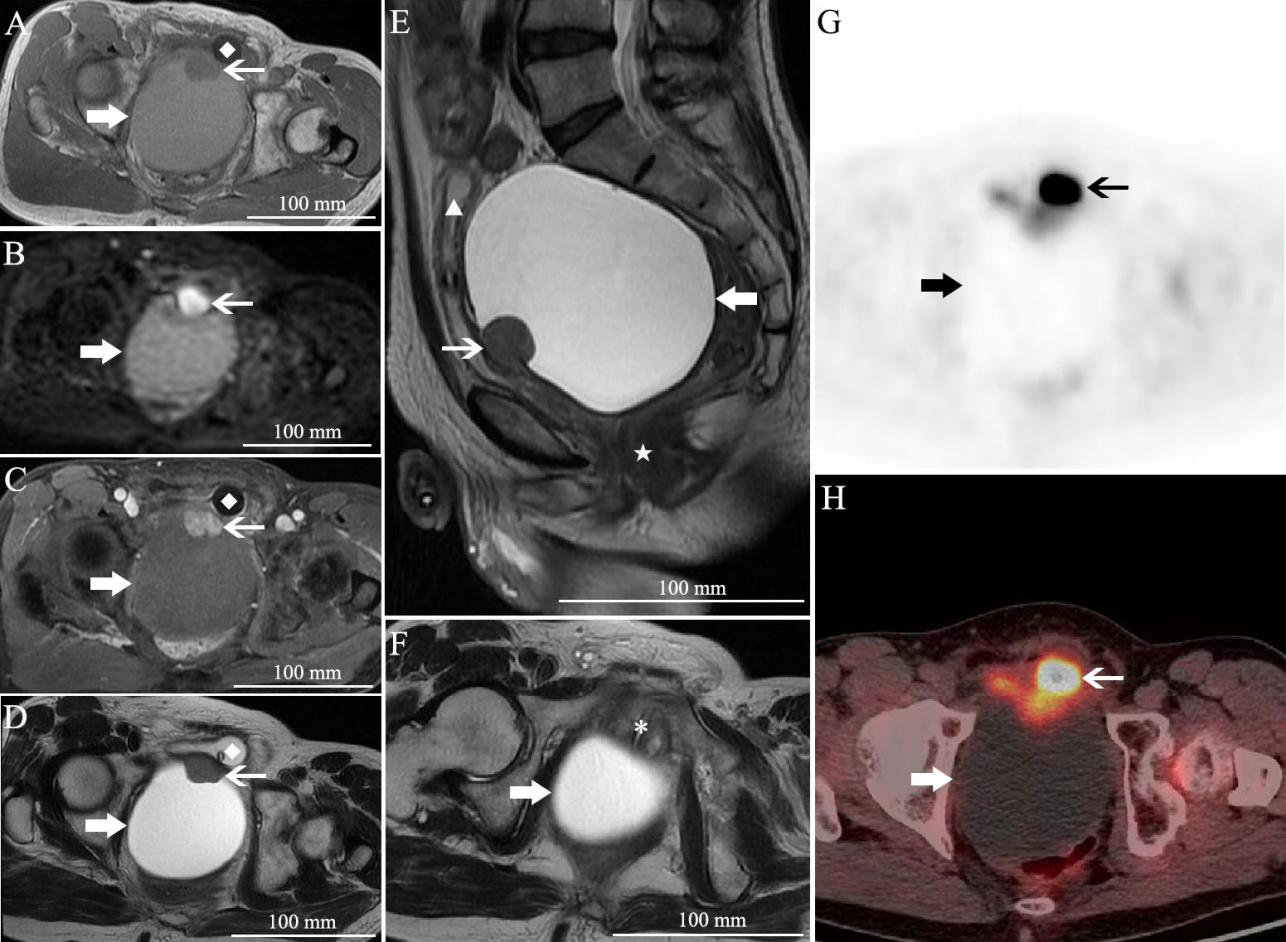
Pelvic MRI and PET/CT. (A) Axial T1 weighted image (WI), (B) axial diffusion weighted image (DWI), (C) axial gadolinium-enhanced fat-saturated T1WI, (D) axial T2WI, (E) sagittal T2WI, and (F) axial T2WI. (G, H)
